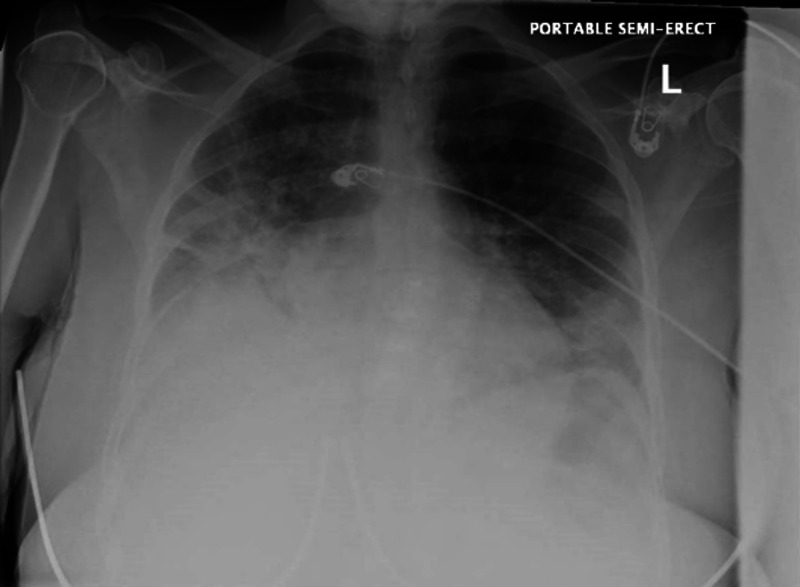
Chest X-ray at presentation to the emergency department showing diffuse predominantly basal and peripheral opacities
